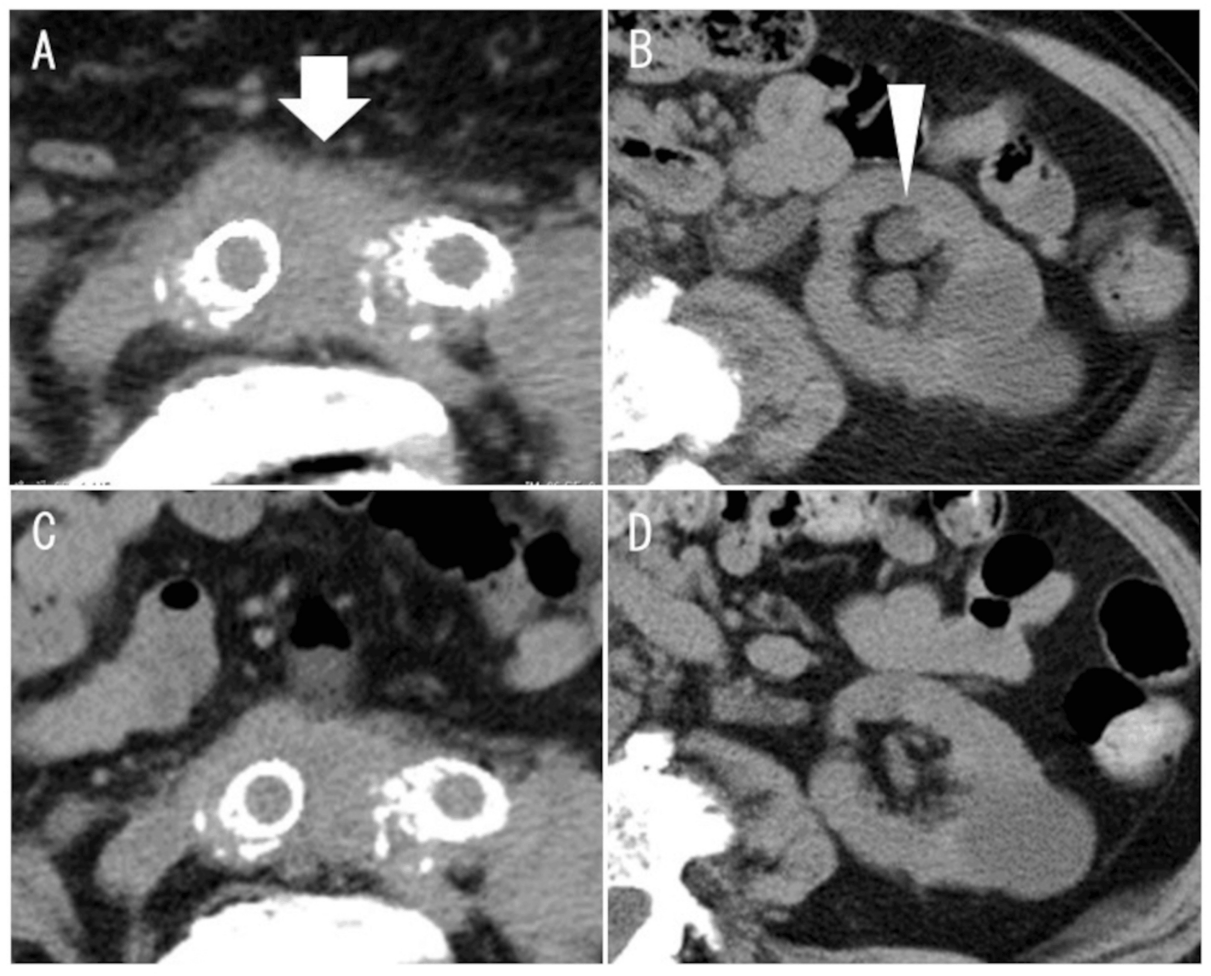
Inflammation recurrence in chronological CT changes CT at postoperative month 12 indicated the recurrence of periarterial thickening (A) (white arrow) and hydronephrosis of the left kidney (B) (white arrowhead), along with steroid reduction. Along with increased steroid dosage, periarterial thickening decreased (C) and hydronephrosis was reduced (D). CT: computed tomography
